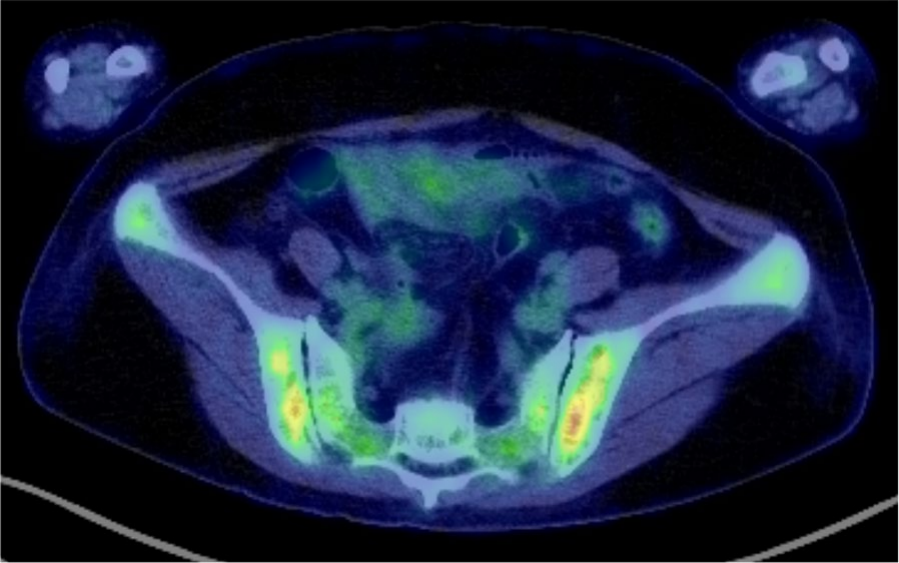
In the PET–CT scan image around the pelvis, diffuse FDG uptake is demonstrated
